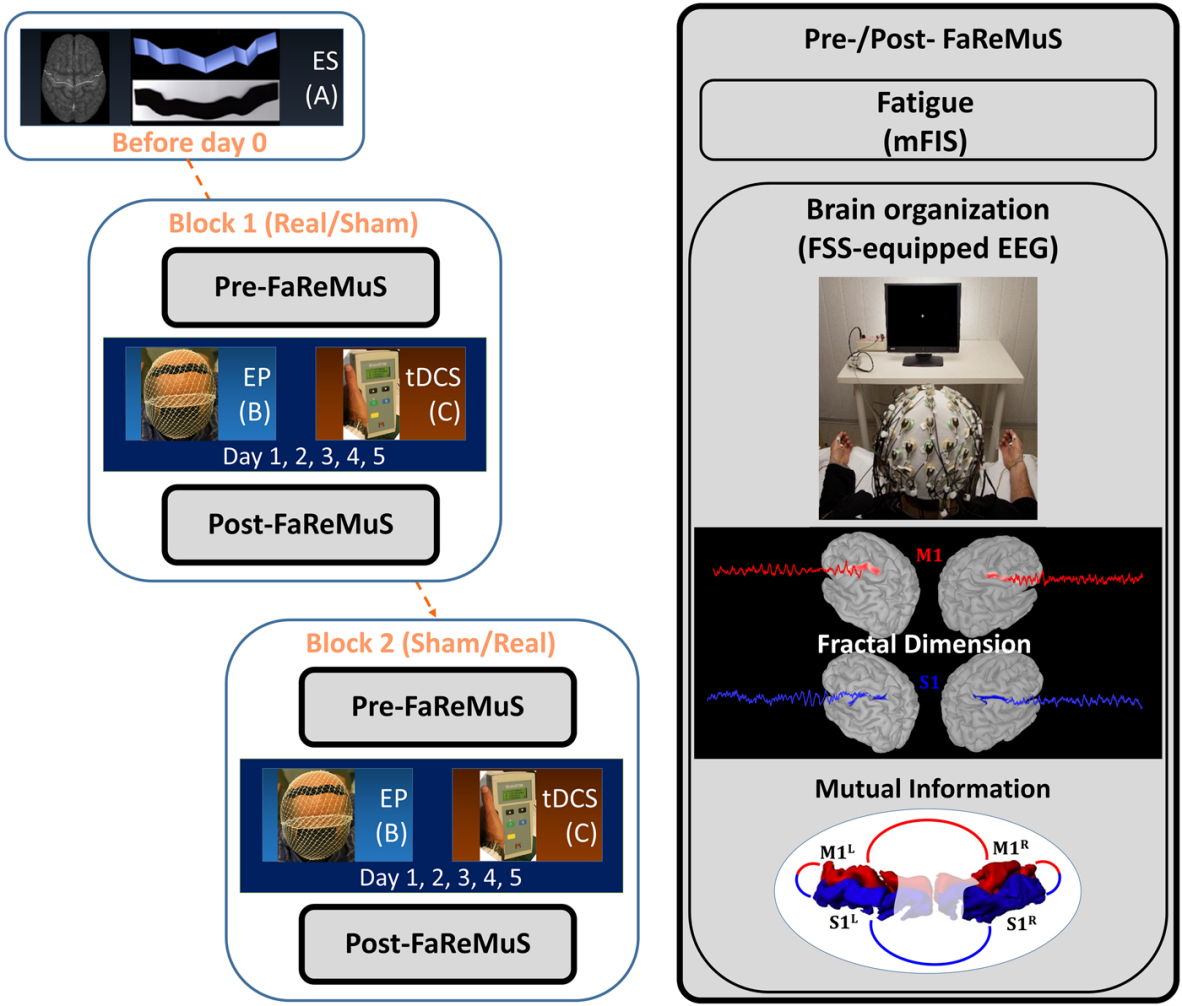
Randomized clinical trial design and FaReMuS effect testing.  Experimental procedure in each person with MS undergoing FaReMuS. Left: Individual brain MRI-based personalized electrode shaping (ES, A) performed once for each person with MS. In blocks 1 and 2 (equal, but each stimulation is either Real or Sham), after the data collection (Pre-FaReMuS), the electrode positioning (EP, B) is executed for each tDCS stimulation (C) repeated for 5 days of treatment. In the day of the last tDCS, we performed the data collection (Post-FaReMuS). Right: The data collection (Pre- and Post-FaReMuS) includes the fatigue level assessment via mFIS and the EEG for the brain effects. We represented the measures used for the resting state local activity (fractal dimension of the primary sensory and motor cortices) and the resting state functional connectivity between them (mutual information).
